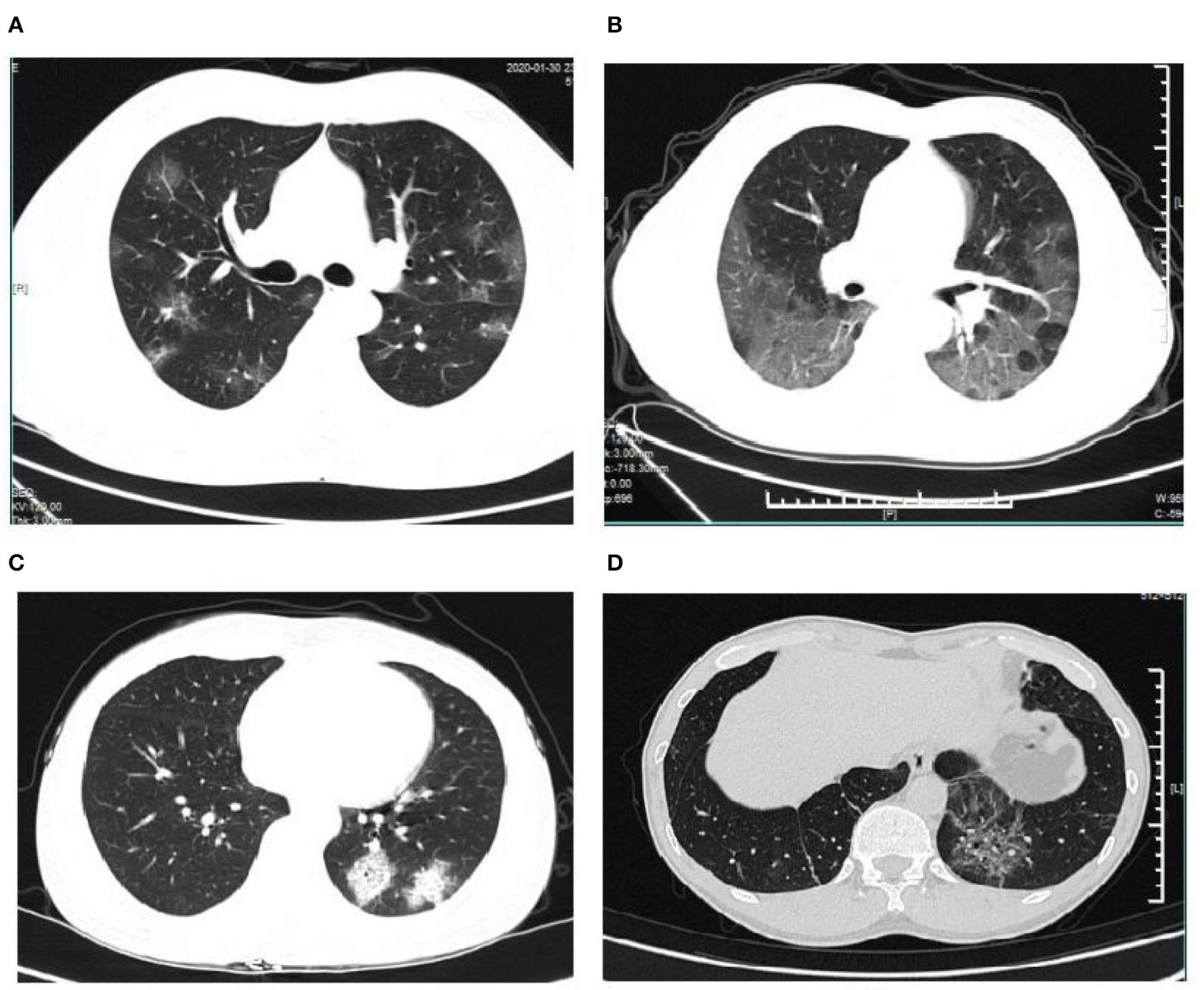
CT-signs of pneumonia in COVID-19 patients. (A) Latticed ground glass sign; (B) white-lung sign; (C) roadstone-like sign; (D) vascular cluster sign.
